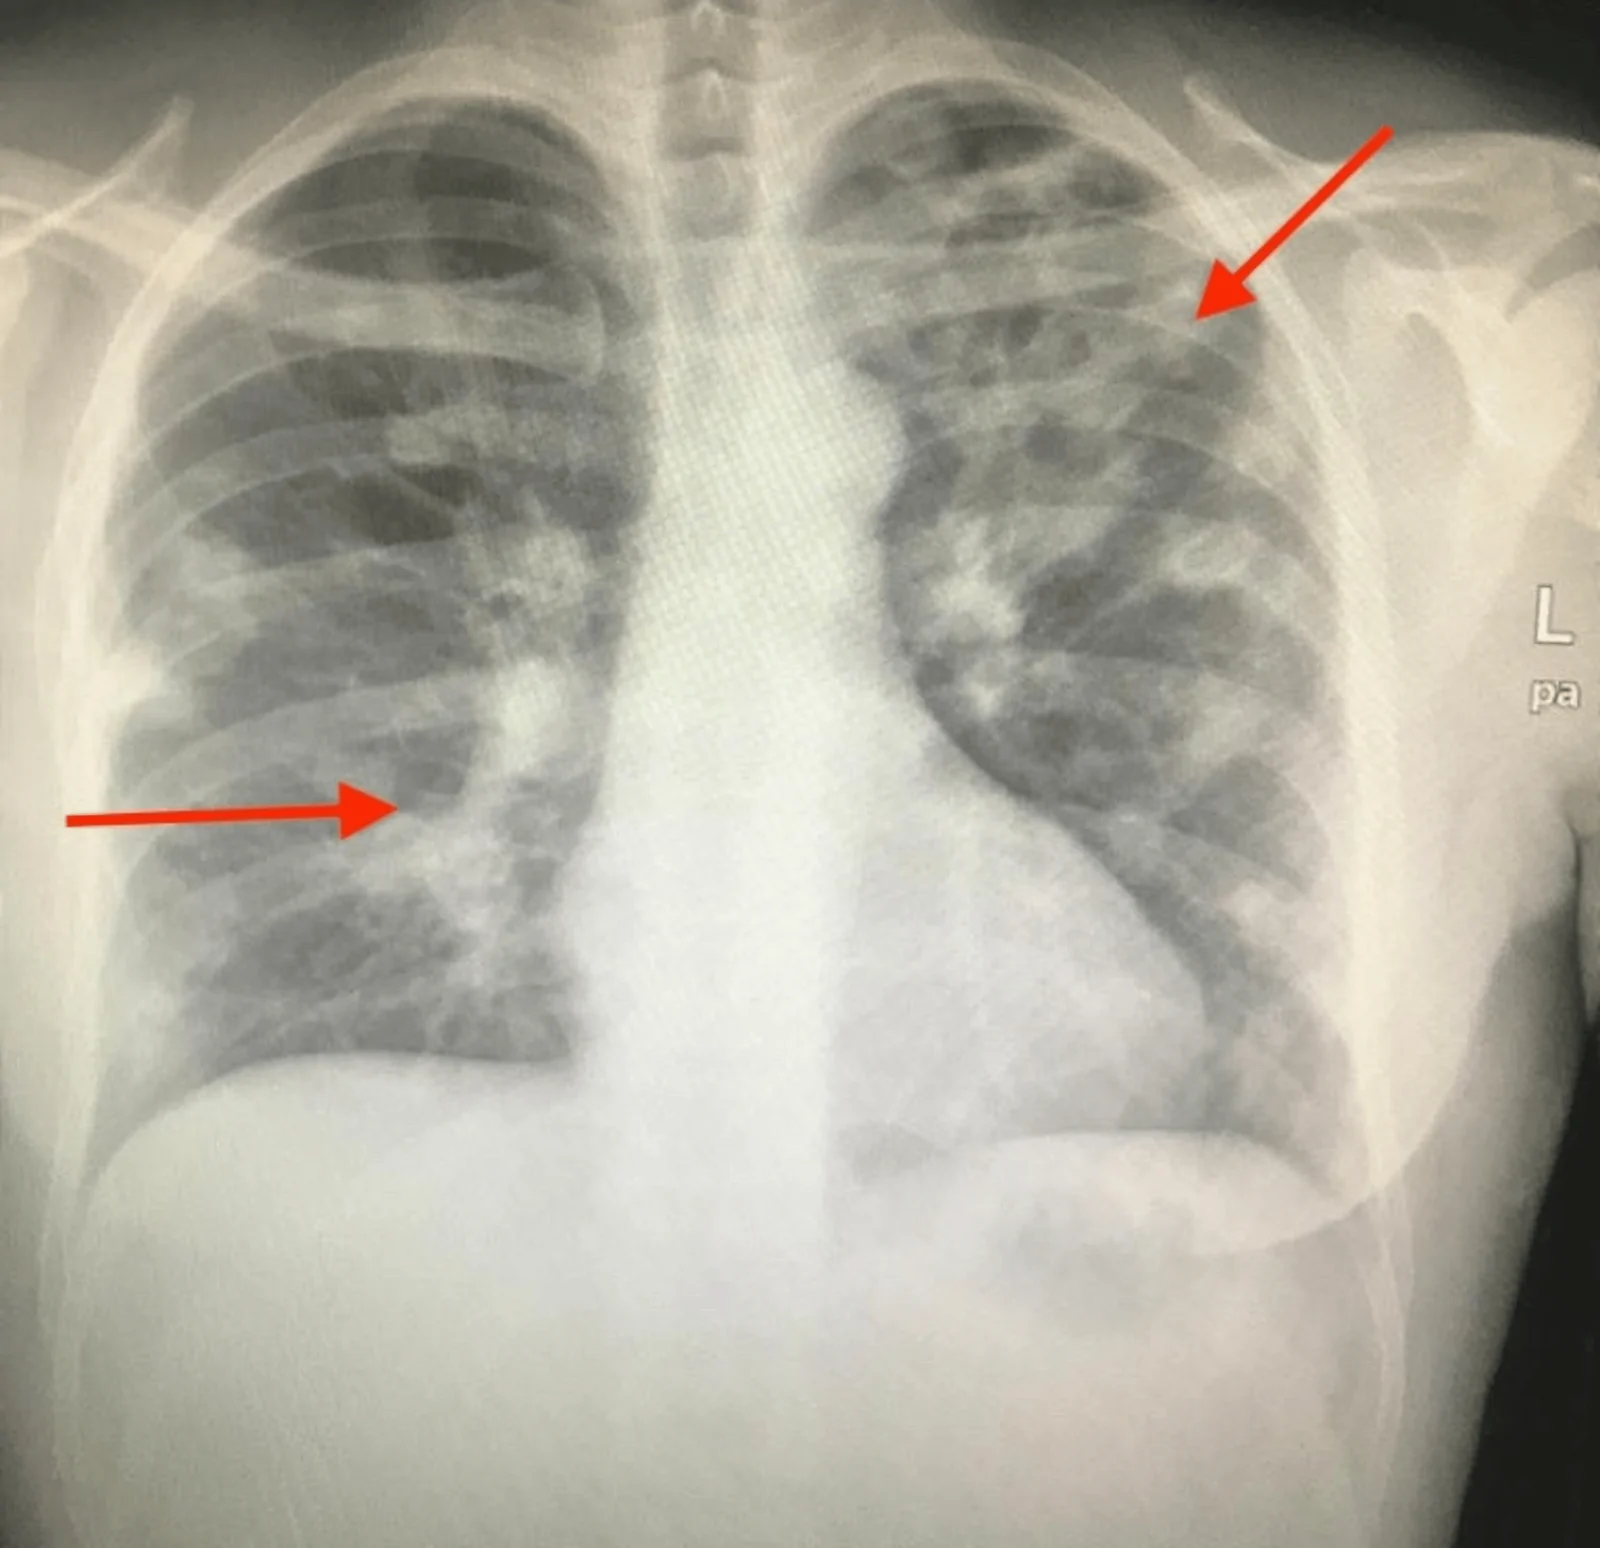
The chest X-ray shows bilateral bronchovascular markings, increased haziness, an obliterated left costophrenic angle, and no pneumothorax.
